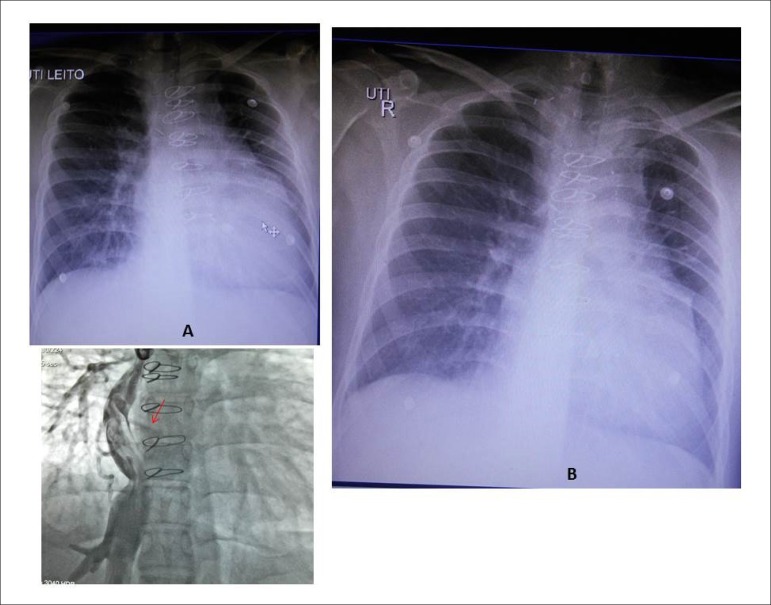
Chest radiographs showing increased heart area, elongated left
